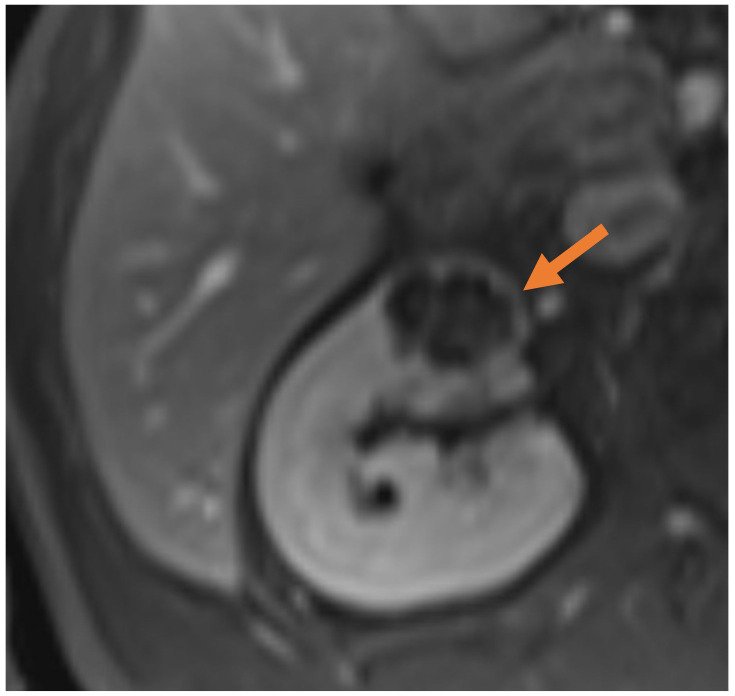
Axial view of contrast-enhanced T1-weighted MRI showing a partly cystic and peripherally enhancing lesion in the anterior right kidney (arrow).
